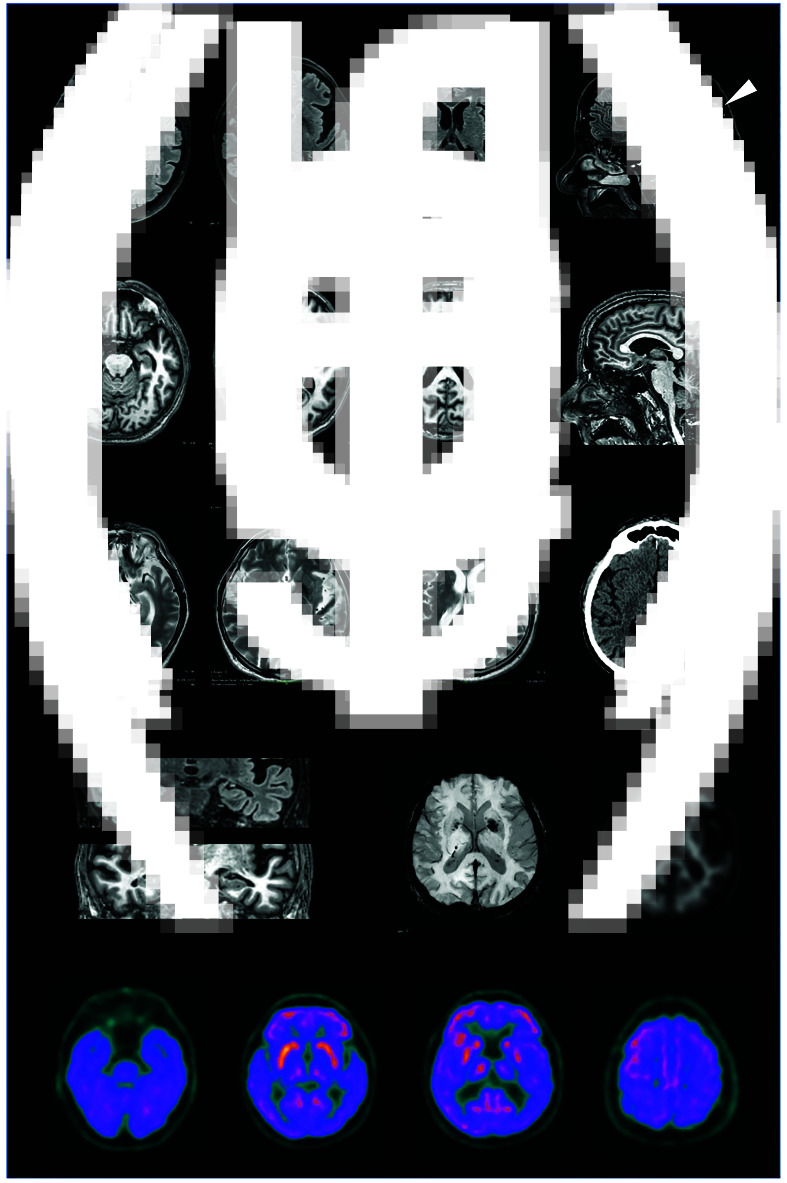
Brain scans showing mild temporo-insular atrophy on both sides, calcifications in the left lenticular nucleus and the location of the previously removed meningioma (arrowhead). (a) Fluid attenuated inversion recovery (FLAIR) and (b) T_1_-weighted axial and sagittal (rightmost images) scans. (c) T_2_-weighted axial scans. (d) Computed tomography axial scan. (e) Coronal FLAIR section of temporal lobes (above) and coronal T_1_ scans of temporal lobes (below). (f) Susceptibility weighted imaging (SWI) scan. (g) Amyloid positron emission tomography (PET) scan, negative for amyloid deposition. (h) fluorodeoxyglucose PET scans showing left superior temporal hypometabolism.
